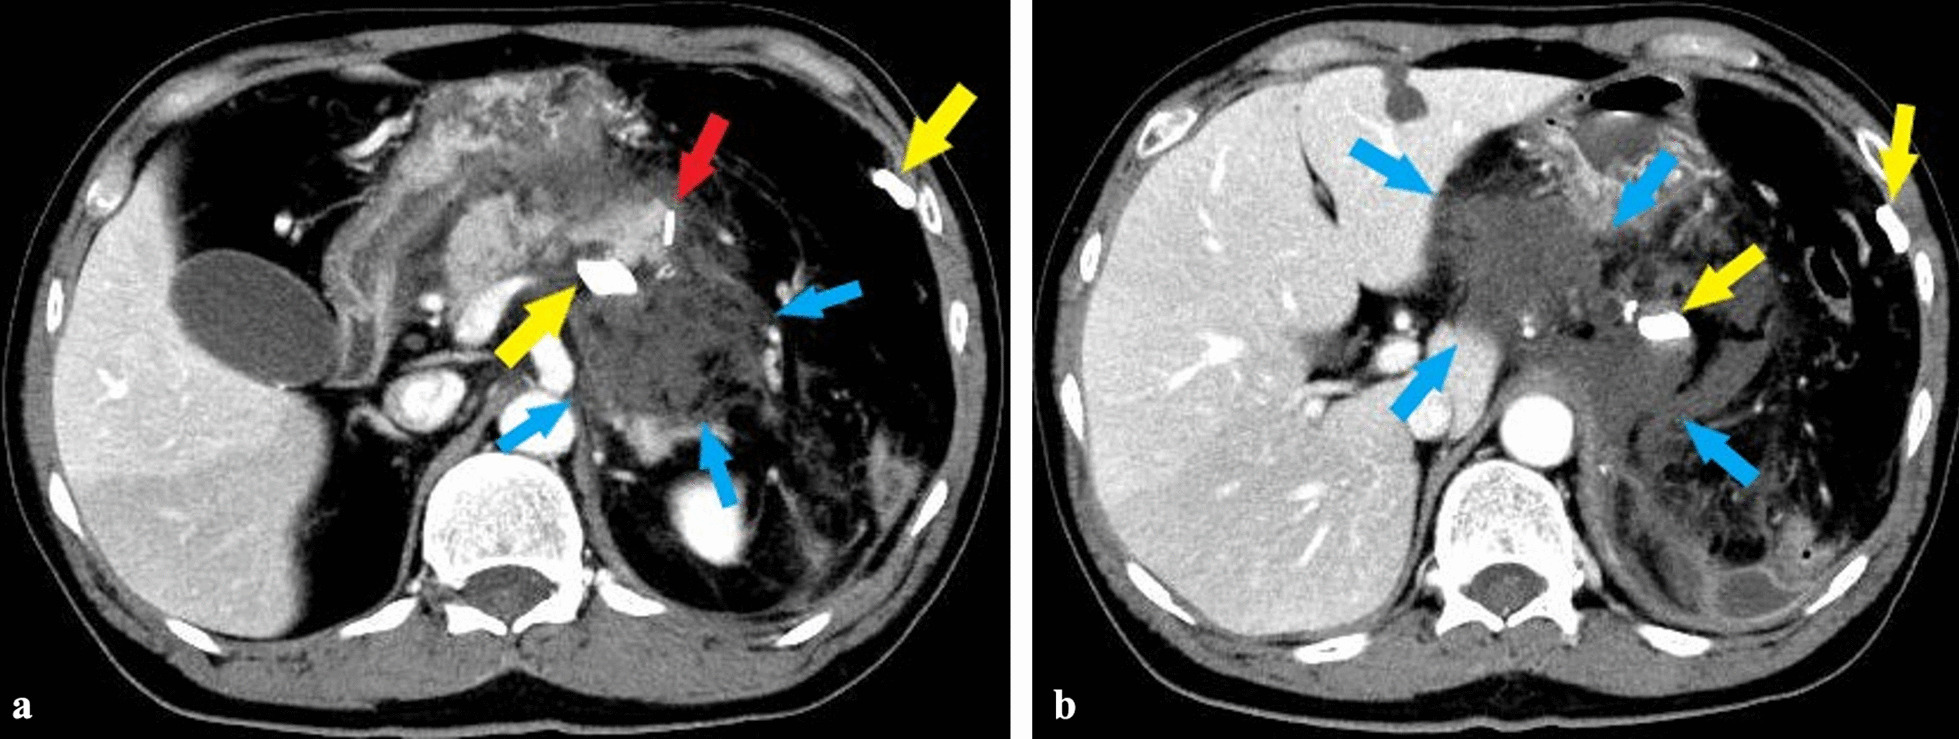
a Contrast-enhanced computed tomography examination on day 7 after surgery. The yellow, red, and blue arrows indicate the drain, stapler of pancreatic stump, and abscess formation of the pancreatic fistula, respectively. b Contrast-enhanced computed tomography examination on day 7 after surgery. The yellow and blue arrows indicate the drain and abscess formation of the pancreatic fistula, respectively
